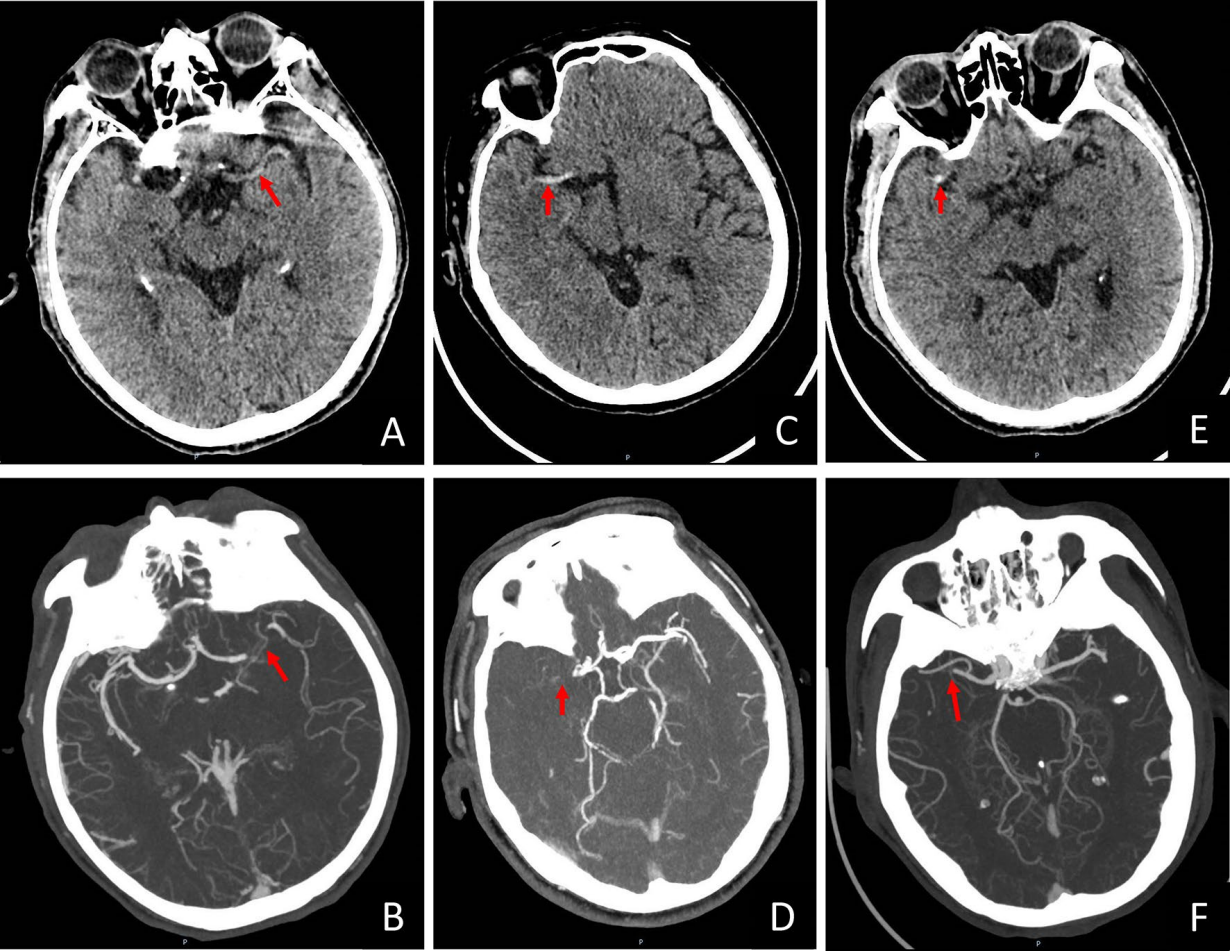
Classification of the hyperdense middle cerebral sign (HMCAS) on no-contrast CT (NCCT) and reconstructed maximal intensity projection (MIP) images. A and B showed the negative HMCAS on NCCT and occlusion of the M1 segment of the middle cerebral artery (MCA) on MIP images in the same patient, respectively. C and D showed the proximal HMCAS on NCCT and occlusion of the M1 segment of the MCA on MIP images in the same patient, respectively. E and F showed a “dot sign” in the sylvian fissure indicating the distal HMCAS on NCCT and occlusion of the M2 segment of the MCA on MIP images in the same patient, respectively
